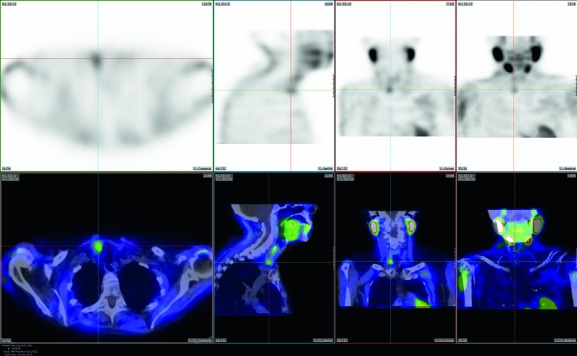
Use of SPECT/CT to aid parathyroid adenoma detection. SPECT-CT images clearly demonstrating a focal area of ^99m^Tc MIBI uptake localized in the lower pole of the right thyroid lobe, as indicated on the (a) axial, (b) coronal, and (c) sagittal imaging planes, as well as the (d) CT, (e) SPECT, and (f) fused data by an arrow. SPECT, single-photon emission computed tomography; CT, computed tomography; ^99m^Tc MIBI, technetium-99m 2-methoxyisobutyl isonitrile. Courtesy of Bankstown-Lidcombe Hospital Nuclear Medicine and Ultrasound Department.
